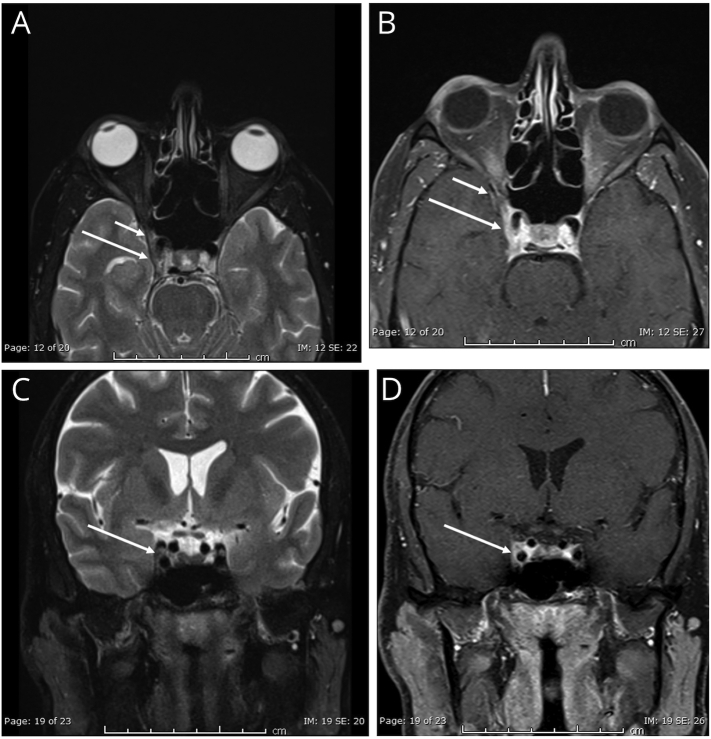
MRI orbits T2 WI, and post I.V. Gadolinium based contrast T1WI, axial orientation (a, and b respectively), and coronal orientation (c, and d respectively), showing T2 intermediate signal and post contrast enhancement increased thickening involving the right cavernous sinus (long arrows in a, b, c, and d), extending to the su*peri*or orbital fissure (short arrows in a, and b).
